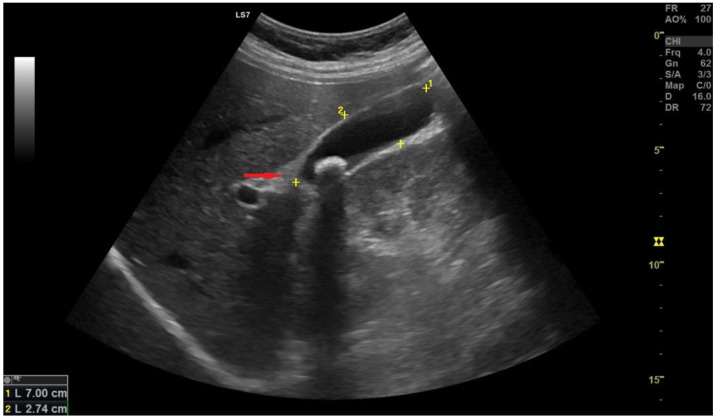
Ultrasonography reveals the site of pancreatic ectopy type 2 (red arrow), opposite the macro calculus with posterior shadow cone.
